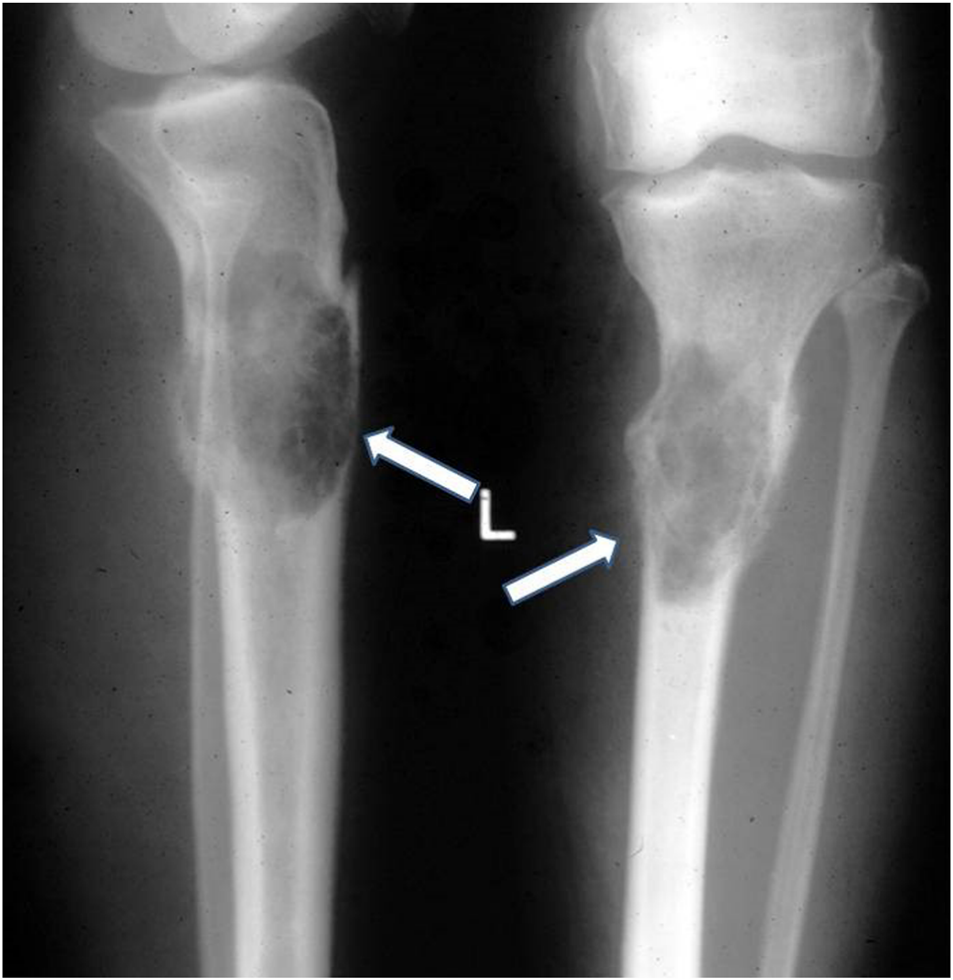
Case 2: x-ray: extensive osteolytic lesion in proximal end of tibia (arrows)
